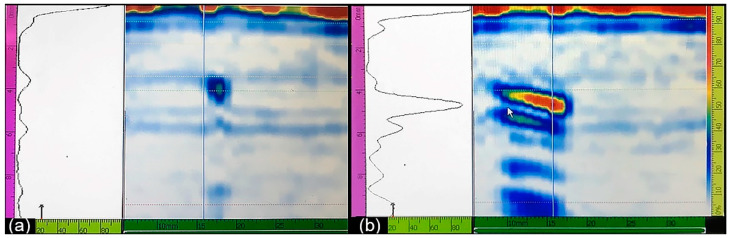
Screenshots from the Olympus OMNISCAN MX2 ultrasonic control box, using the Dual Linear Array 7.50L32-REX1-IHC probe, showing (a) the initial crack of approximately 0.5 mm length and (b) the same crack at the final stage with the transverse length of 10 mm.
